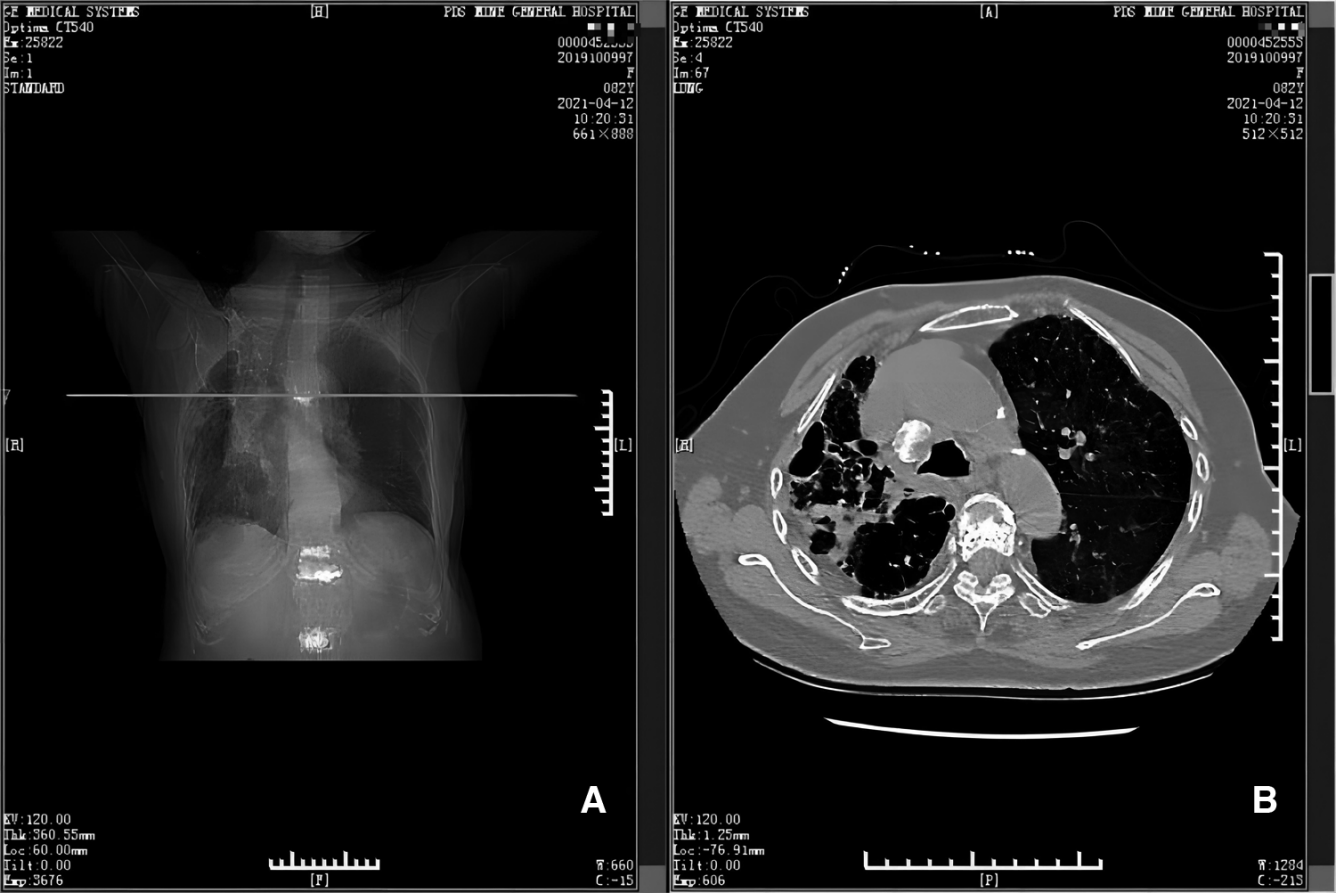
CT scan taken at 17th month postoperatively (panels A and B). Panel A depicts the position (horizontal line) of the CT scan shown in B, at the level of the 5th thoracic vertebra. Panel B shows there is no hematoma near the 5th thoracic vertebra and adjacent ribs.
